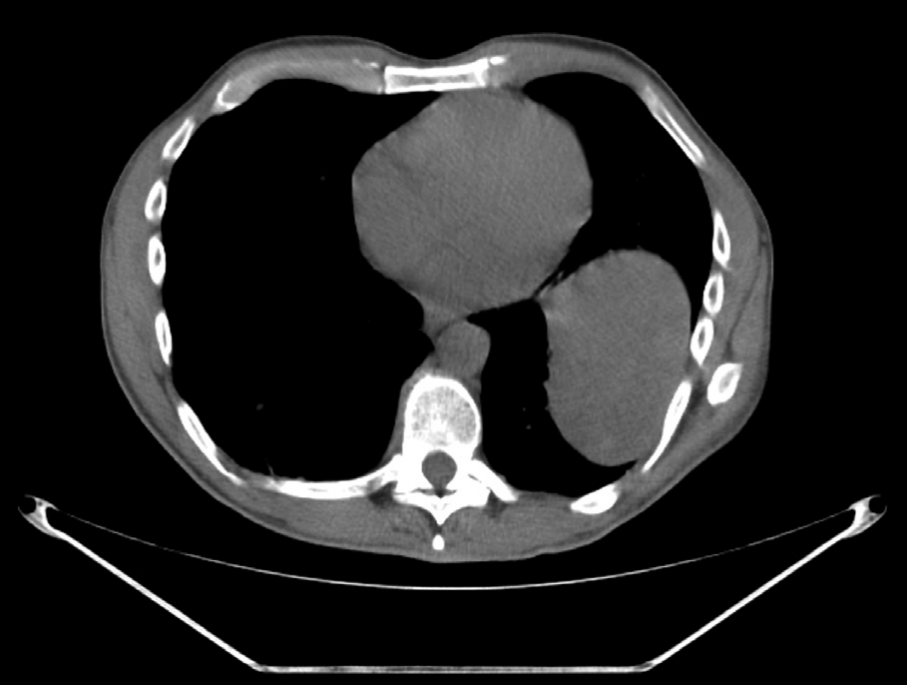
Computed tomographic scan revealing a large pleural-based mass in the left hemithorax.
